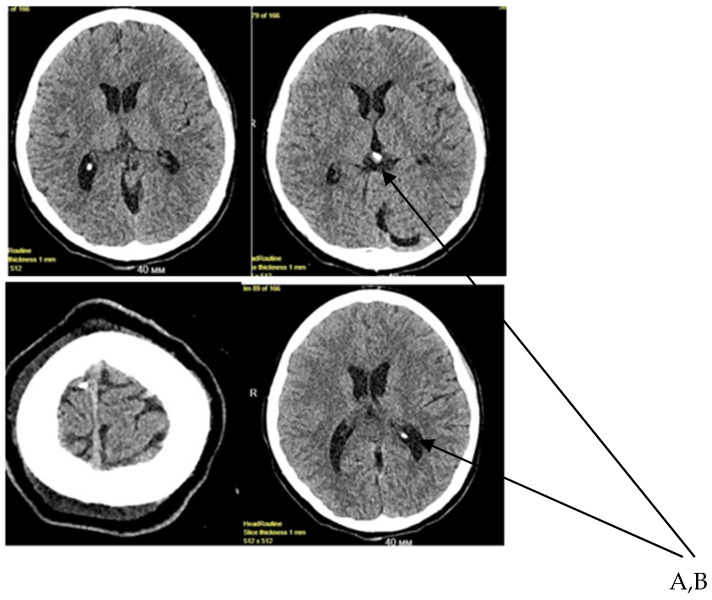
Brain MRI demonstrating a ring-enhancing lesion in the cerebellar hemisphere (arrow), associated with perifocal edema and compression of the fourth ventricle ((A,B)—calcified foci in the brain).
